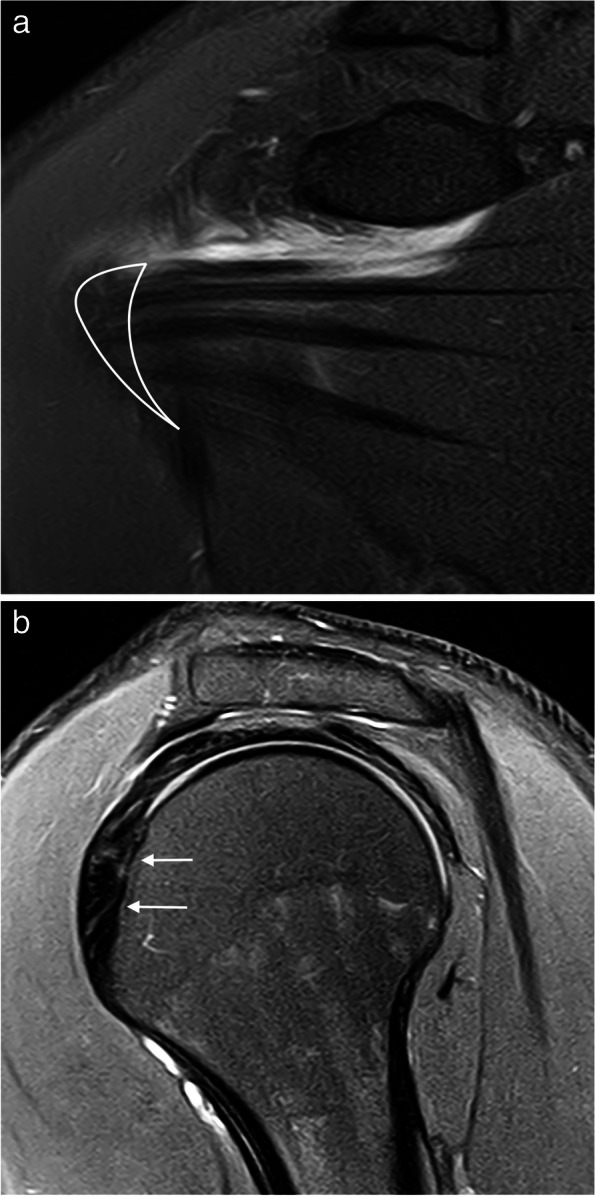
Normal subscapularis. a Oblique coronal fat-suppressed PD-weighted MRI showing the comma shape of the SSC insertion (white outline). b Oblique sagittal fat-suppressed PD-weighted FS MRI demonstrate the normal appearance of the tendon (arrows)
